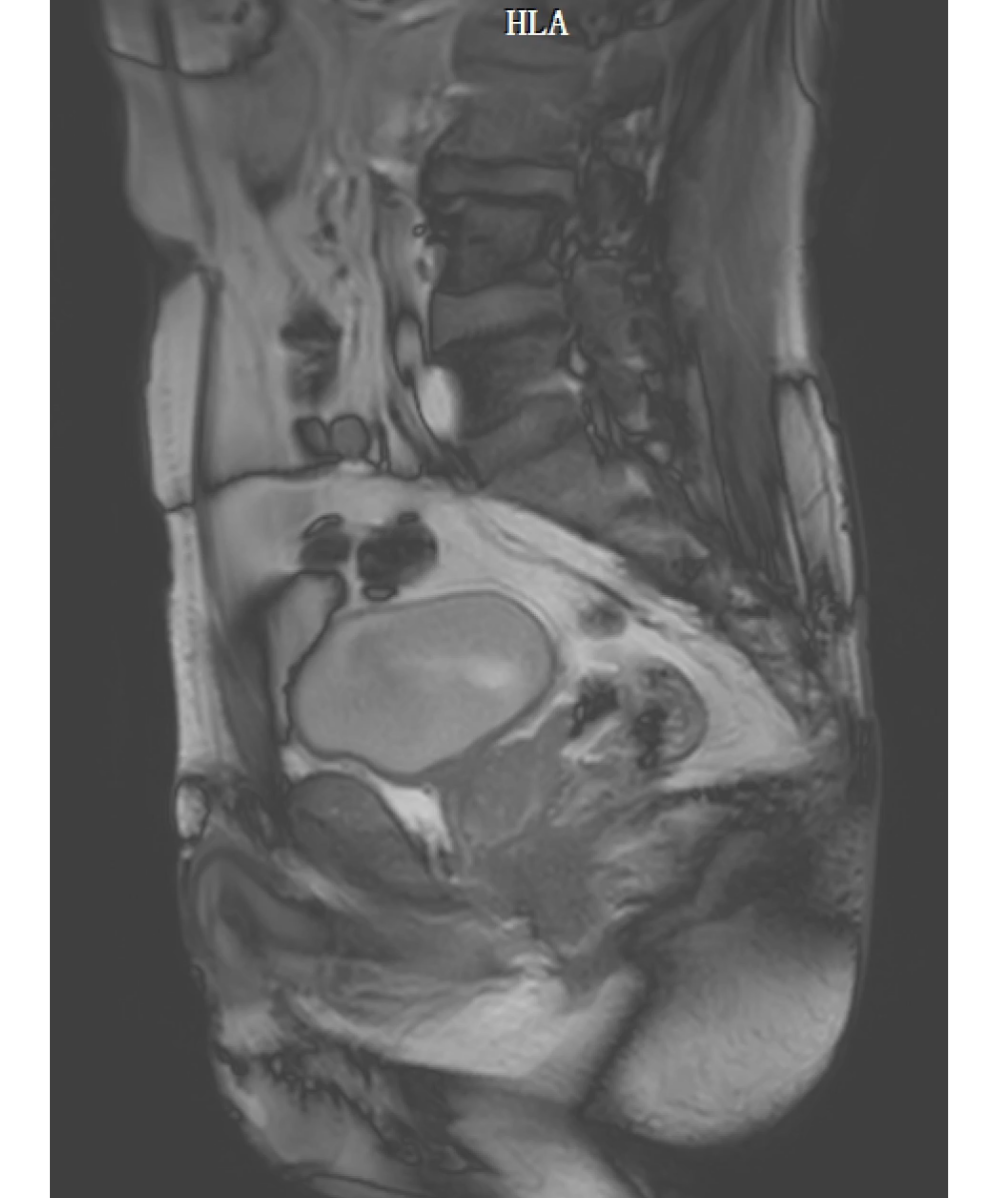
Computed tomography image of the older male participant. HLA: head left anterior.
